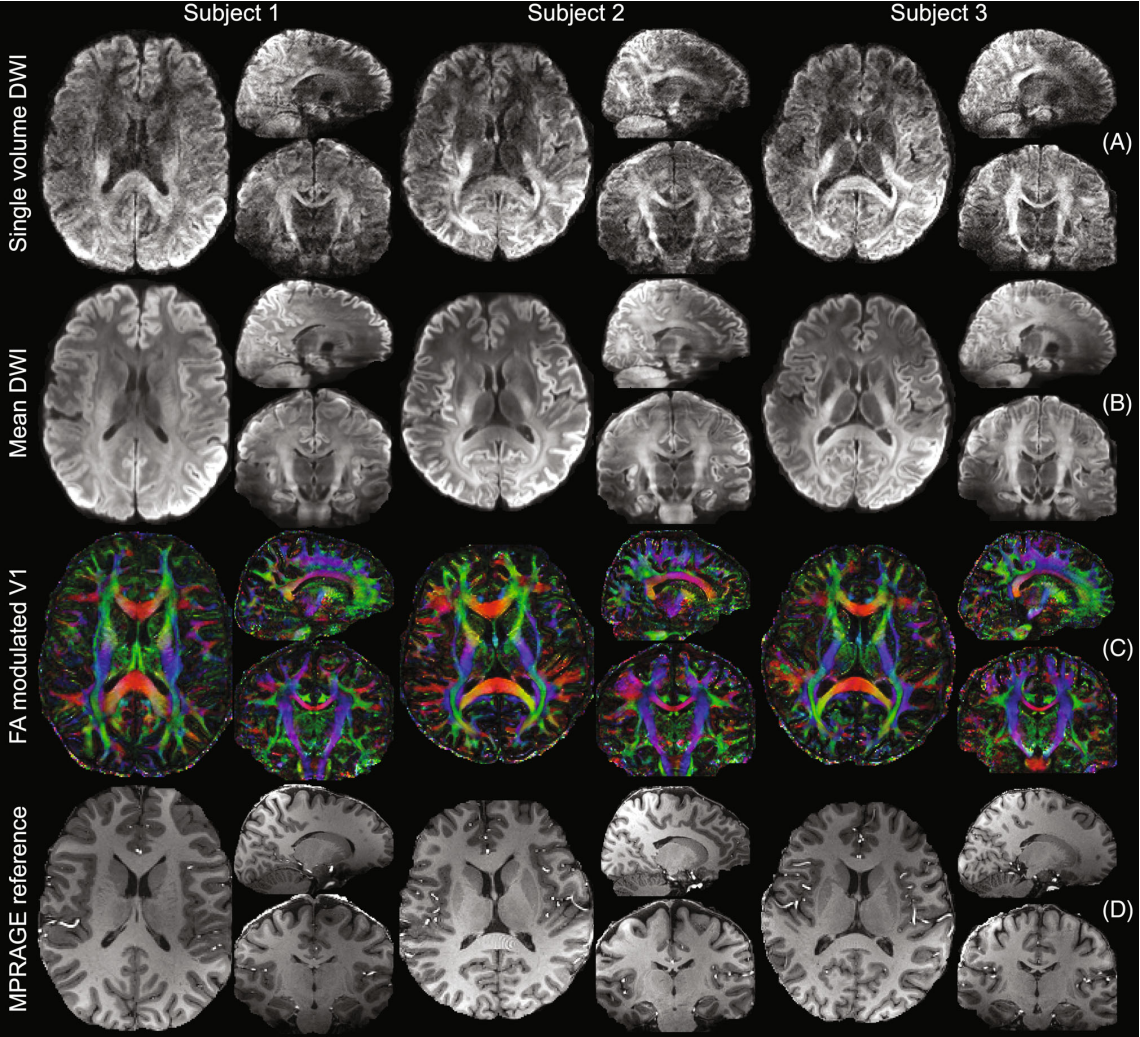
Diffusion MRI results of multiple subjects using the proposed method. The single volume DWI along the diffusion direction (−0.26, −0.81, −0.52) (A), the mean DWI (B), and the fractional anisotropy (FA) modulated primary eigenvector (V1) of diffusion tensor (C), and the anatomical images for reference (D) of three subjects are displayed. The diffusion images are acquired with the high‐resolution protocol at 1.05 mm isotropic resolution using joint blip‐up/down with CAIPI‐PF acquisition. The anatomical images are acquired with MPRAGE at 0.86 mm isotropic resolution and co‐registered to diffusion space for comparison.
